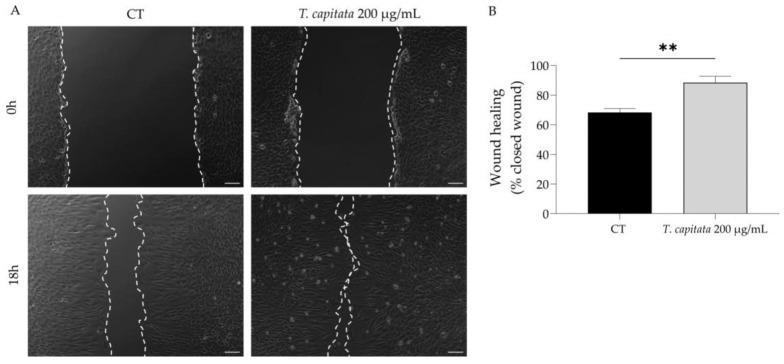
Effect of *T. capitata* extract on the wound healing of NIH/3T3 fibroblasts. Representative bright-field images of NIH/3T3 fibroblasts 0 h and 18 h (A) and percentage of closed wound (B) of NIH/3T3 fibroblasts 18h after scratch wound assay, in the presence (gray bar) or absence (black bar) of the extract. Results show the mean ± standard error of the mean (SEM) of at least three independent experiments.  *p* < 0.01 when compared to CT after unpaired Student’s *t*-test. Scale bar: 100 µm.
